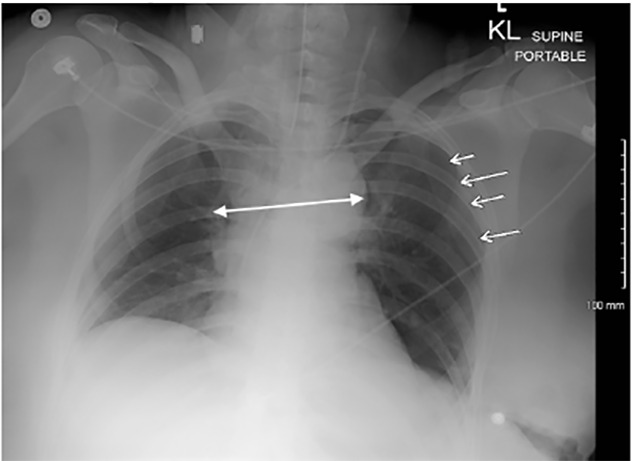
Anteroposterior supine chest radiograph revealing slight mediastinal widening (double-headed arrow), pneumothorax (arrows), and multiple left-sided rib fractures.
